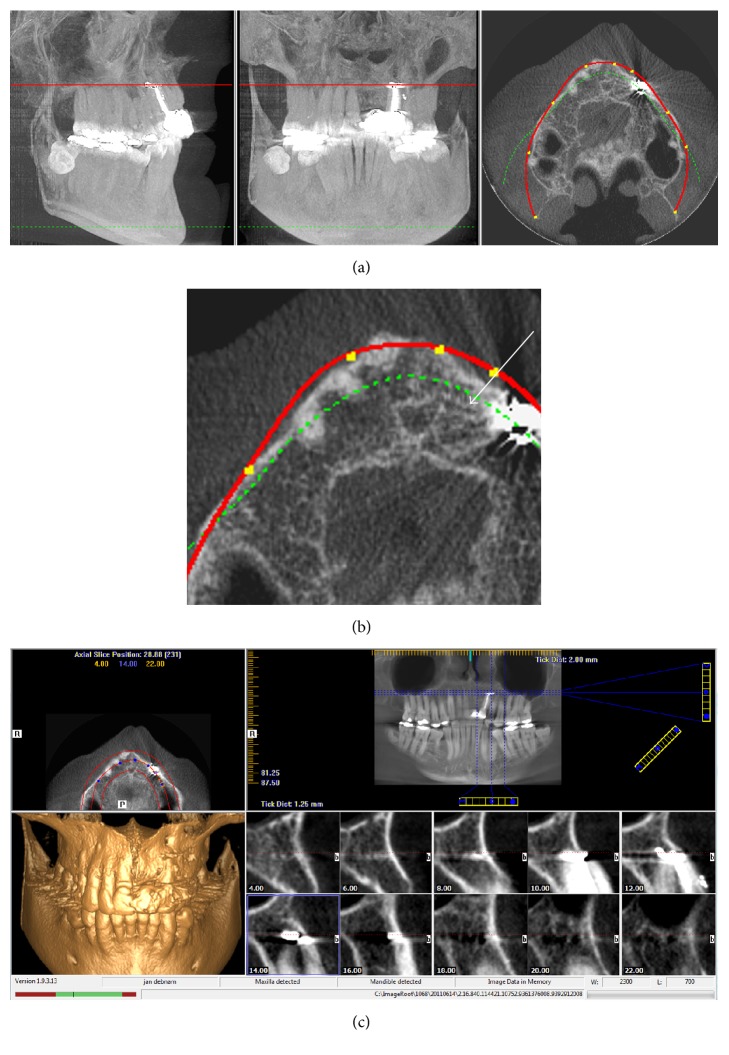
(a) CBCT (iCAT) imagery taken during original implant assessment by implant clinic. (b) Magnified axial image from (a) showing that a well-demarcated canal is present between the apex of tooth 23 and the nasopalatine canal, white arrow. (c) Multiple foramina are easily seen throughout the apical/nasal region prior to implant placement.
